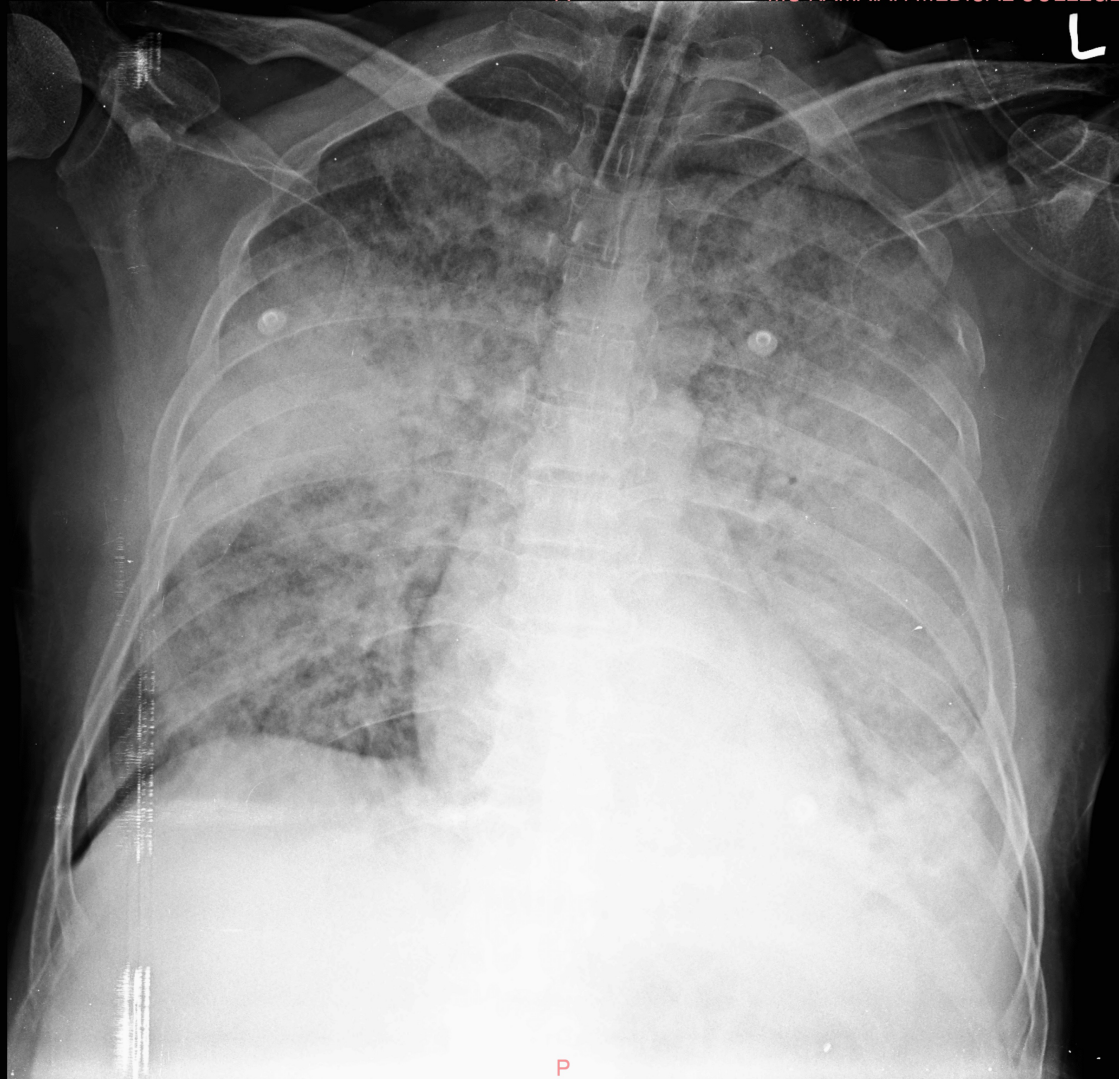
Chest X-ray demonstrating a worsening non-cardiogenic pulmonary edema consistent with ARDS ARDS: Acute respiratory distress syndrome
